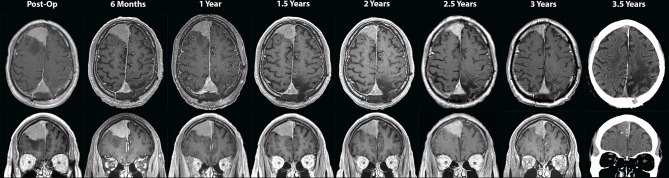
Serial axial (top panels) and coronal (bottom panels) T1-weighted post-contrast MRI images taken every 6 months during outpatient follow-up, including the initial post-operative scan showing parasagittal parietal meningioma invading the superior sagittal sinus left as residual tumor. Follow-up scans at 6 months, 1 year, and 1.5 years show that the two lesions remained stable in size. On 2-year through the most recently taken 3.5-year scans, regression of both the left parasagittal parietal and the (untreated) right frontal lesions is visualized and attributed to the abscopal effect. A CT scan was performed for follow-up at 3.5 years due to recent placement of a non-MRI-compatible cardiac pacer.
